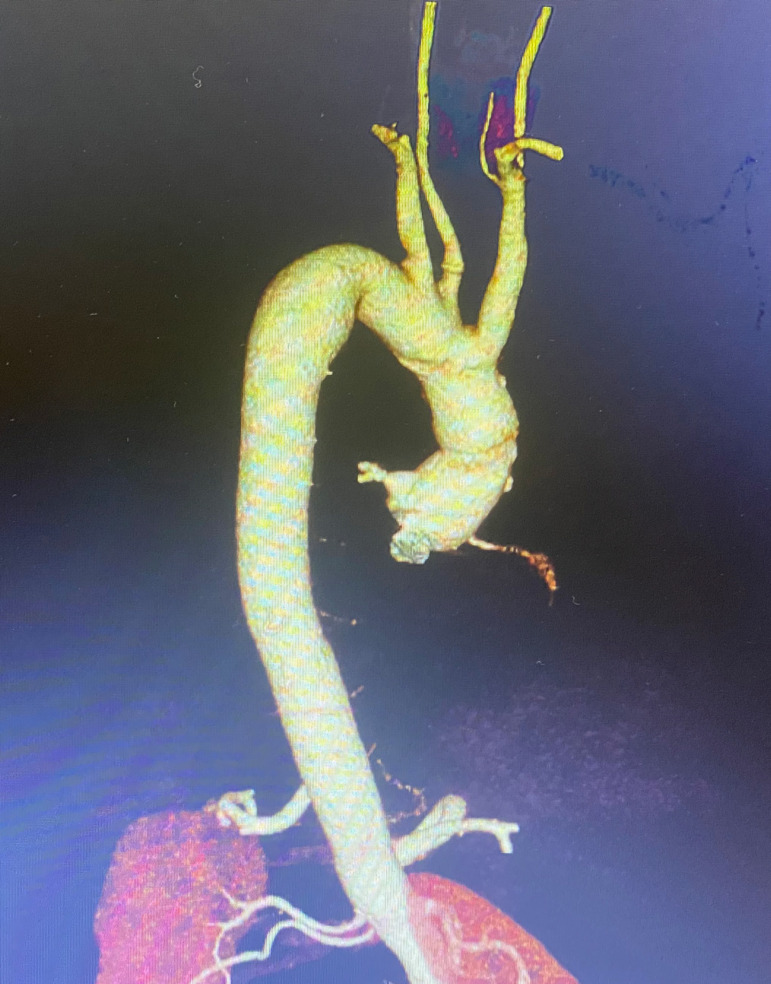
Postoperative 3D computed tomography image of the patient after total
